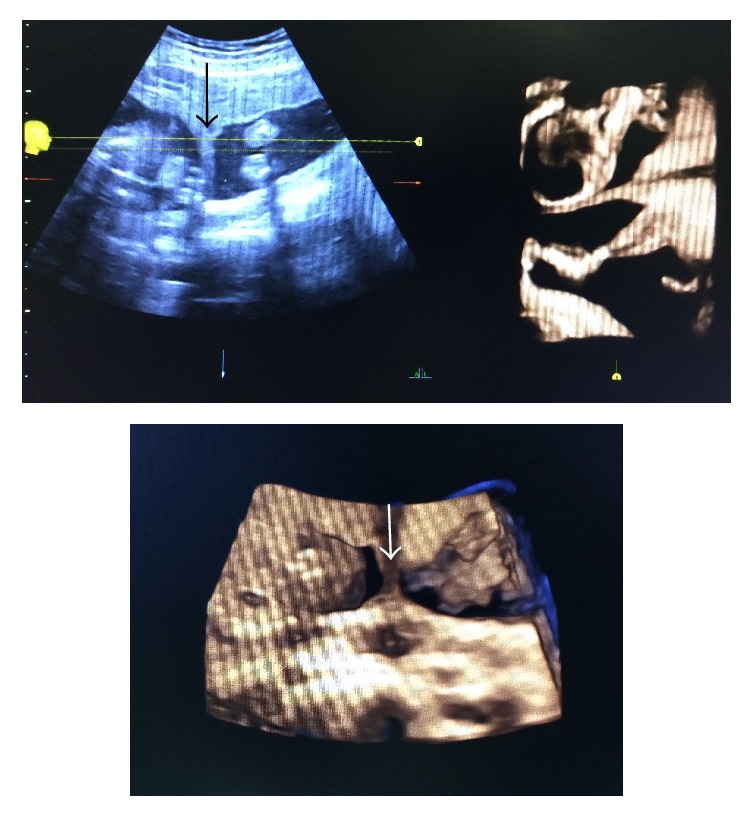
Thick septum separating the uterine cavities showing in 2D and 3D ultrasound mode. The arrows showed thick septum separating the uterine cavities showing in 2D and 3d ultrasound mode.
